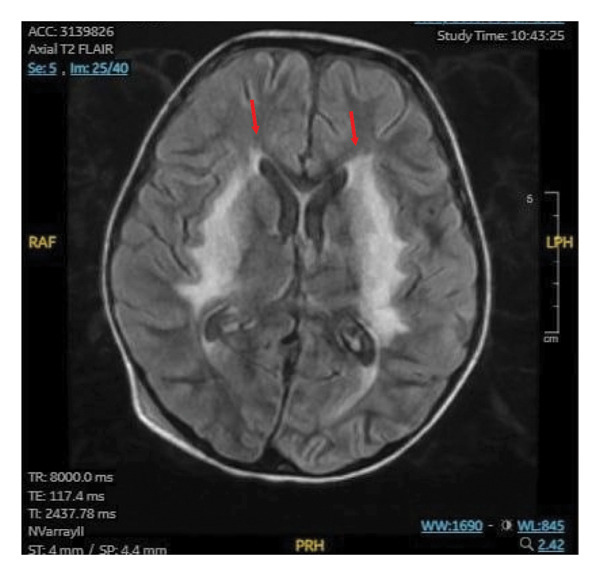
Confluent periventricular and subcortical FLAIR and T2WI hyperintense signal abnormalities were observed in the frontal and parietal lobe white matter. Furthermore, the external and extreme capsules, claustrum, and subinsular white matter bilaterally with few foci of cortical changes involving the right frontal lobe showed FLAIR and T2WI hyperintense signal changes.
